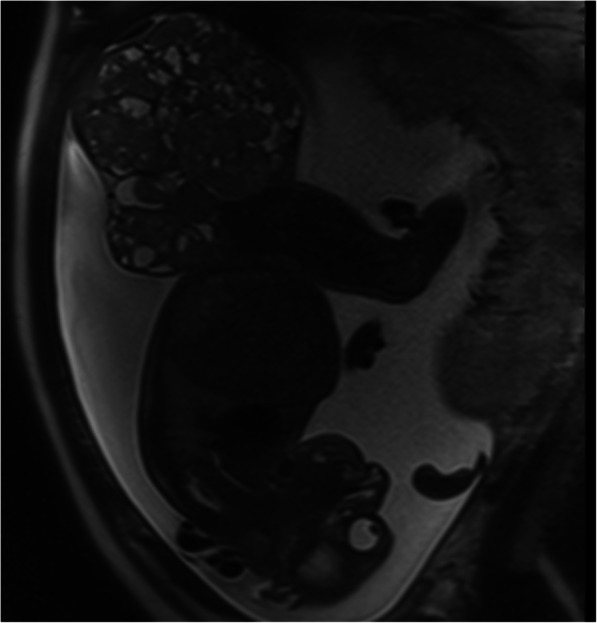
Follow-up MRI at 29 weeks where it’s seen the growth of the sacrococcygeal teratoma
